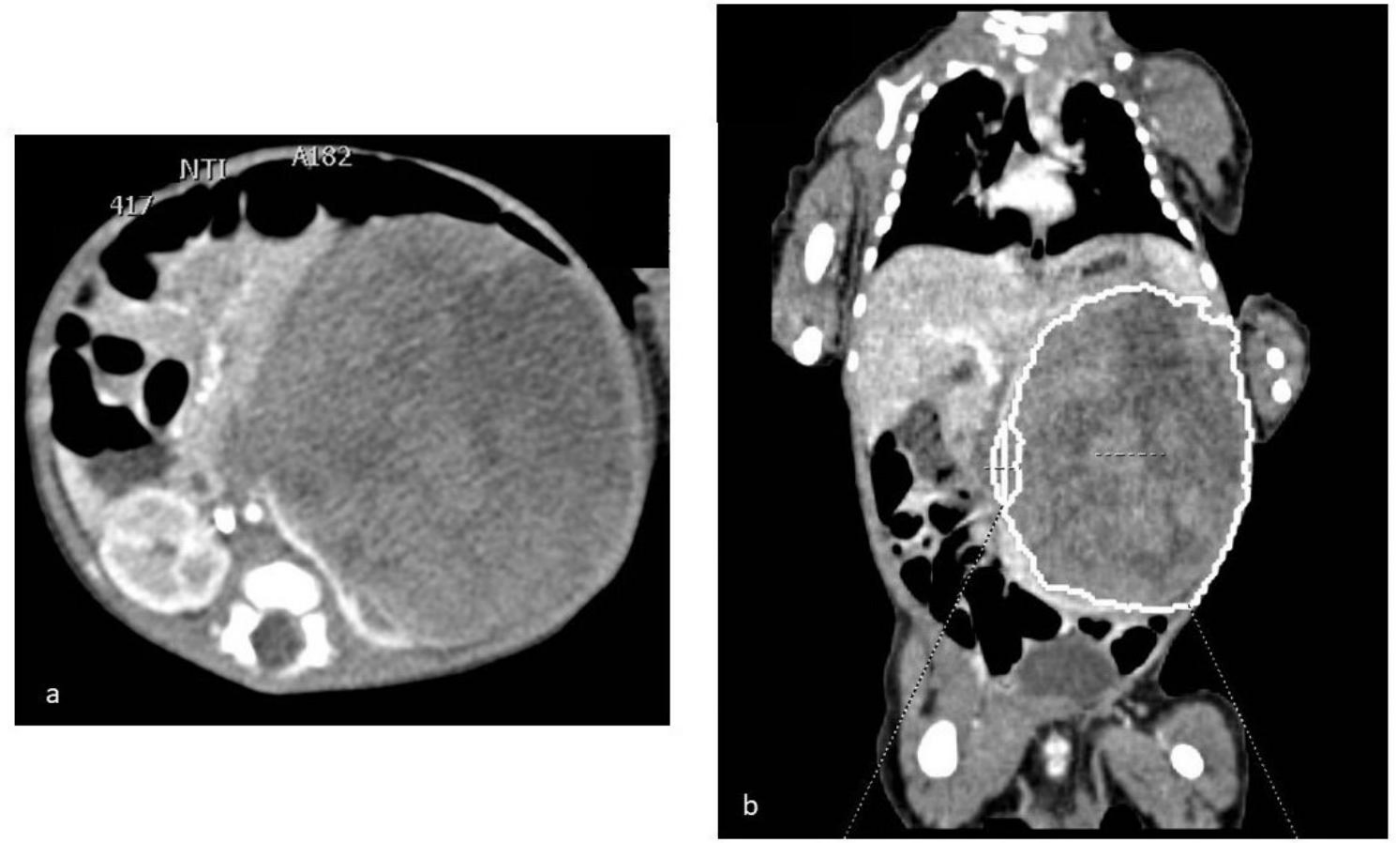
Patient 2. Abdominal (a) and chest (b) CT shows a mass in the left renal lodge, without lymph node enlargement/infiltration or secondary pleuropulmonary lesions
